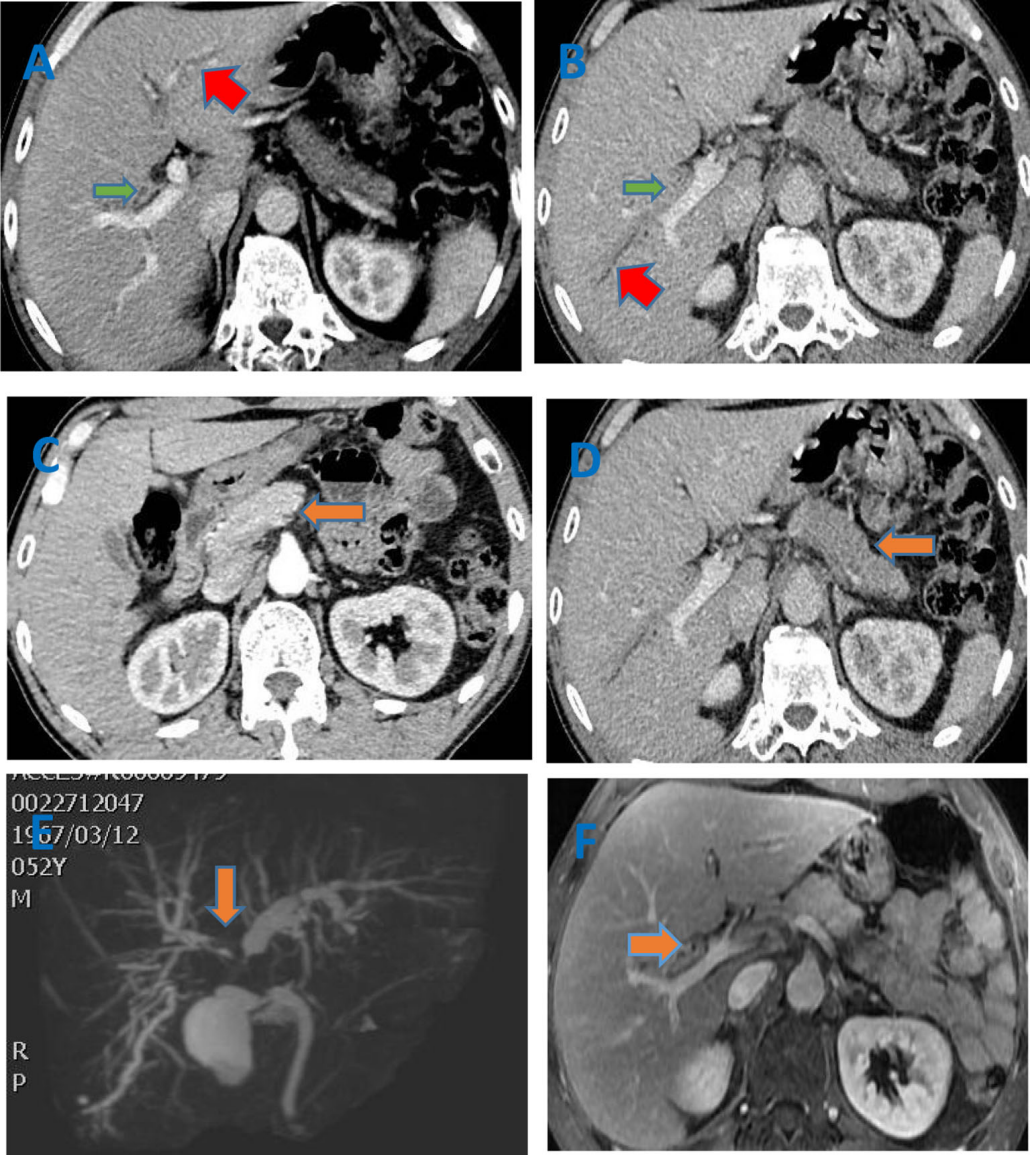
(A–D) Enhanced CT of the abdomen showed that the common hepatic duct, the beginning of the left hepatic duct (red arrow), the right hepatic duct (green arrow) and its branch walls were thickened and gradually strengthened. The intrahepatic bile ducts were slightly dilated. The pancreas (orange arrow) was full, and the edges were irregular. After the enhancement, the enhancement at the arterial phase decreased and showed low density. But it further enhanced with the delay. No pancreatic duct dilation was seen; (E) Biliary MRCP indicated multi-segment lumen stenosis of the bile duct. The thickening of the tube wall involved a large extent, which did not match the degree of lumen expansion. The thickening of the tube wall was obvious, irregular, and asymmetric; (F) The enhanced MRI of liver indicated that the bile duct wall was gradually strengthened. The pancreas was full with straight contour. The T2 signal was increased,and the TI signal was reduced. The enhancement was uniform. The pancreatic duct was not dilated.
